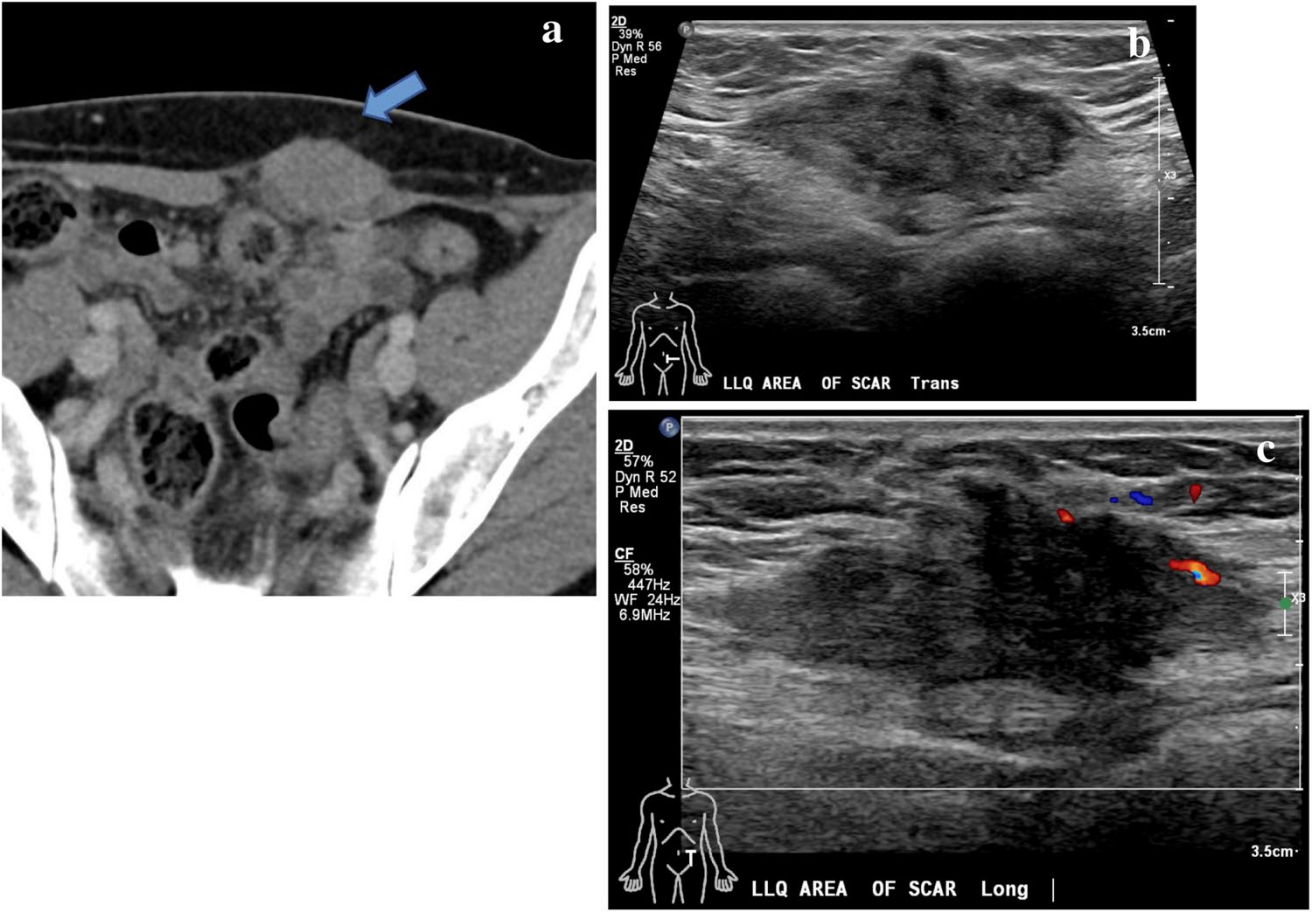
Thirty-six-year-old female with history of Cesarean section and an endometrioma implant of the abdominal wall. Postcontrast axial CT (a) of the pelvis demonstrates a homogeneously enhancing oval mass within the left rectus abdominis muscle. Corresponding targeted grayscale (b) and Doppler (c) ultrasound demonstrates an avascular, heterogeneously hypoechoic soft tissue mass with irregular margins measuring 4.6 cm along the site of Cesarean section scar
